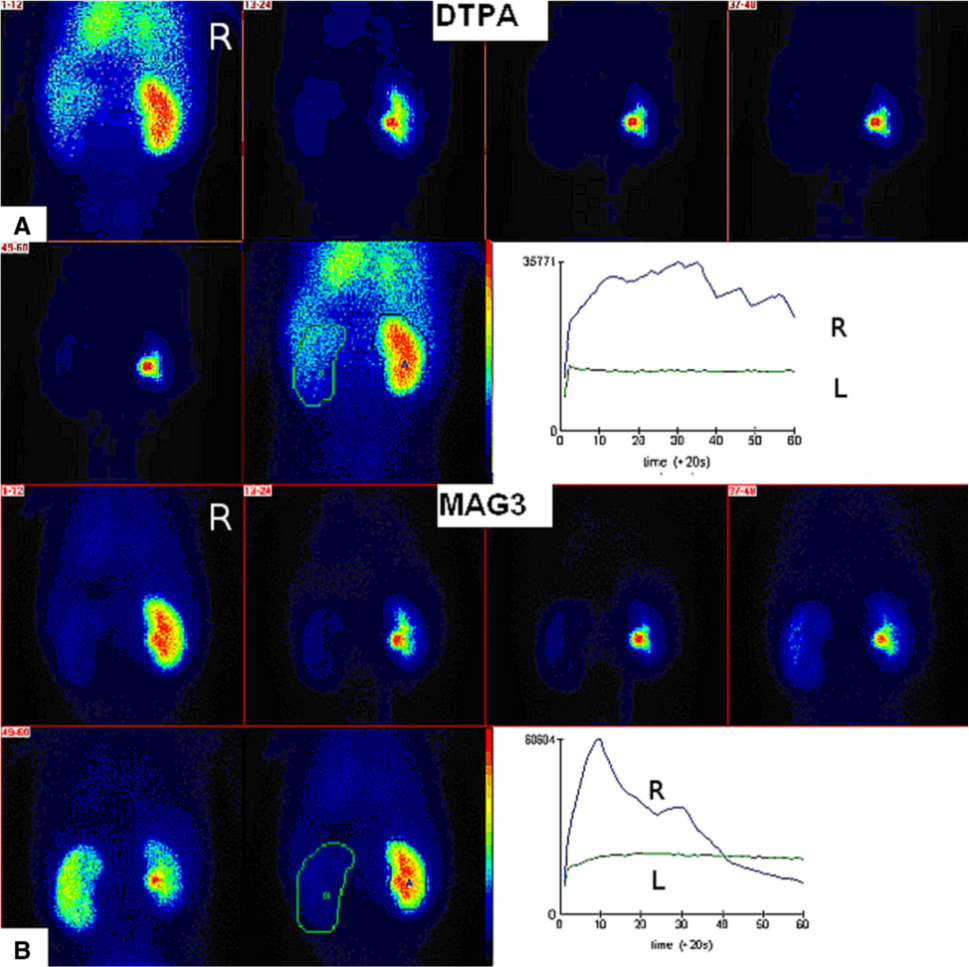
Renal imaging in posteroanterior position (R = right kidney, L = left kidney). A. 99mTc-DTPA renal scintigraphy shows vascular bed over the left kidney without visualization of the parenchyma with practically afunctional renographic curve of the same kidney. B. 99mTc-MAG3 scintigraphy shows very pale left kidney becoming increasingly better visualized later. Renographic curve of the left lidney shows obstruction over the third phase of the renogram.
